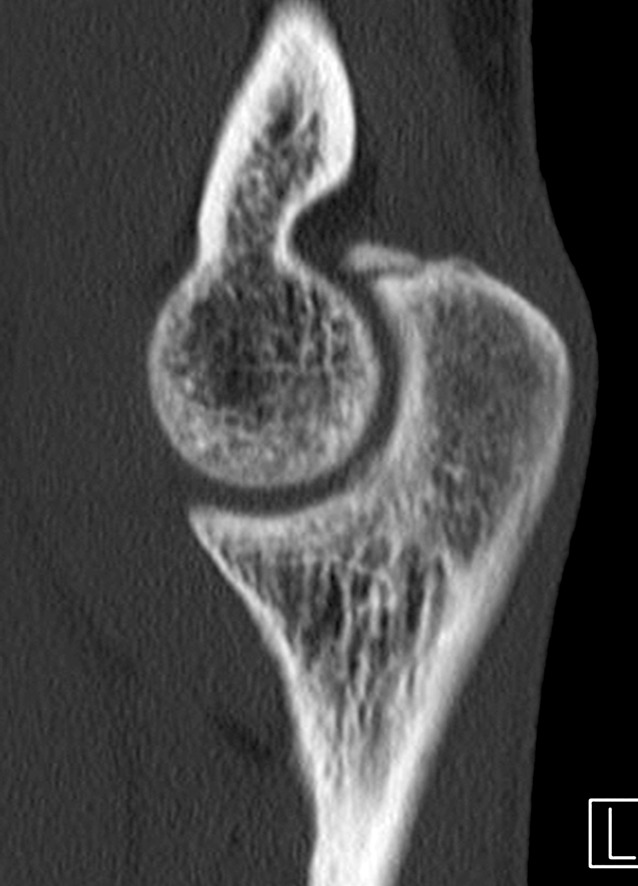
Computed tomography scan of the elbow showing a fractured osteophyte at the tip of the olecranon. (Courtesy of MoRe Foundation)
